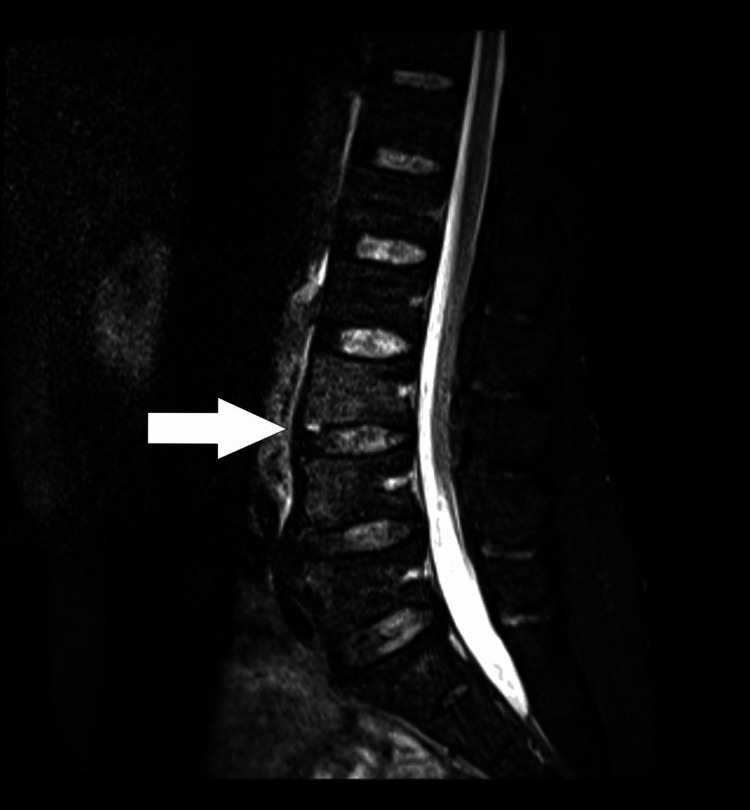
Sagittal MRI with STIR sequence demonstrating mild vertebral body edema at the L3 and L4 levels concerning for discitis-osteomyelitis (arrow). MRI: magnetic resonance imaging; STIR: short tau inversion recovery
